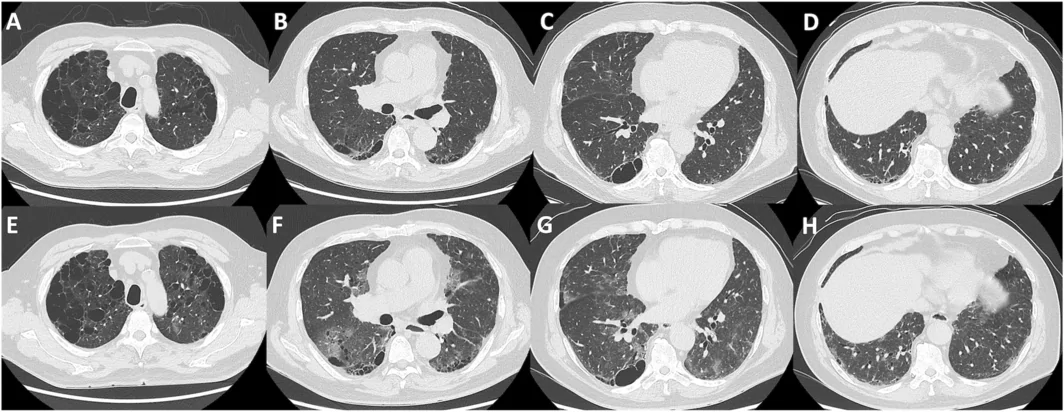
High‐resolution computed tomography (HRCT) images obtained 2 weeks before admission and at admission. (A–D) Scans obtained 2 weeks before admission showing no apparent new infiltrates. (D–H) Scans at admission demonstrating multiple newly developed bilateral ground‐glass opacities.
